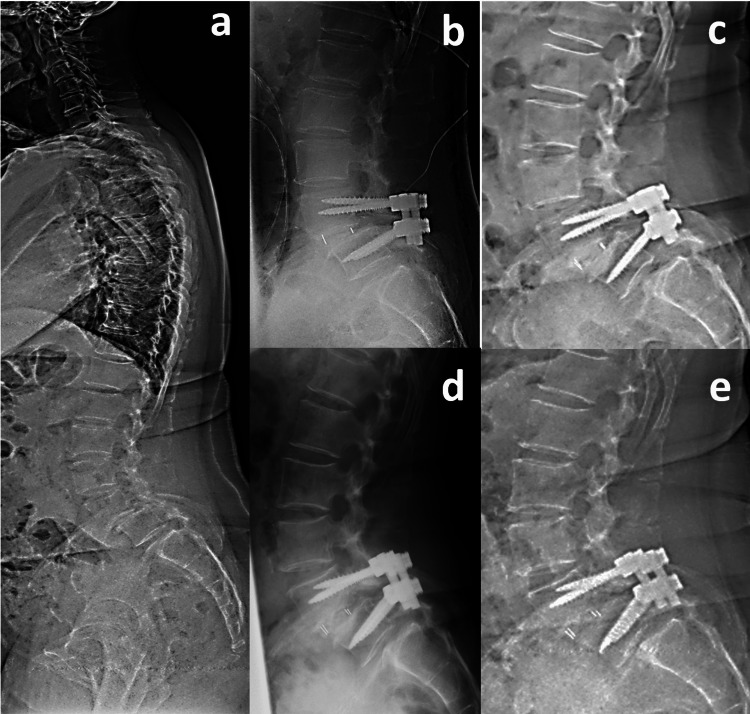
Pre and postoperative radiographs of Case 2 a. Preoperative sagittal whole-spine radiograph: LL 61˚, PI 78˚, PT 30˚, SVA 93 mm, L4 segmental lordosis 22˚, and L4-SVA 64 mm. b. Immediate postoperative lateral lumbar radiograph: L4 segmental lordosis 28˚. c. Lateral lumbar radiograph at fracture onset showing newly developed L5 spondylolisthesis: LL 60˚ and L4-SVA 65 mm. d. Lateral lumbar radiograph after early ambulation. e. Lateral lumbar radiograph at final follow-up demonstrating no further progression of spondylolisthesis.
